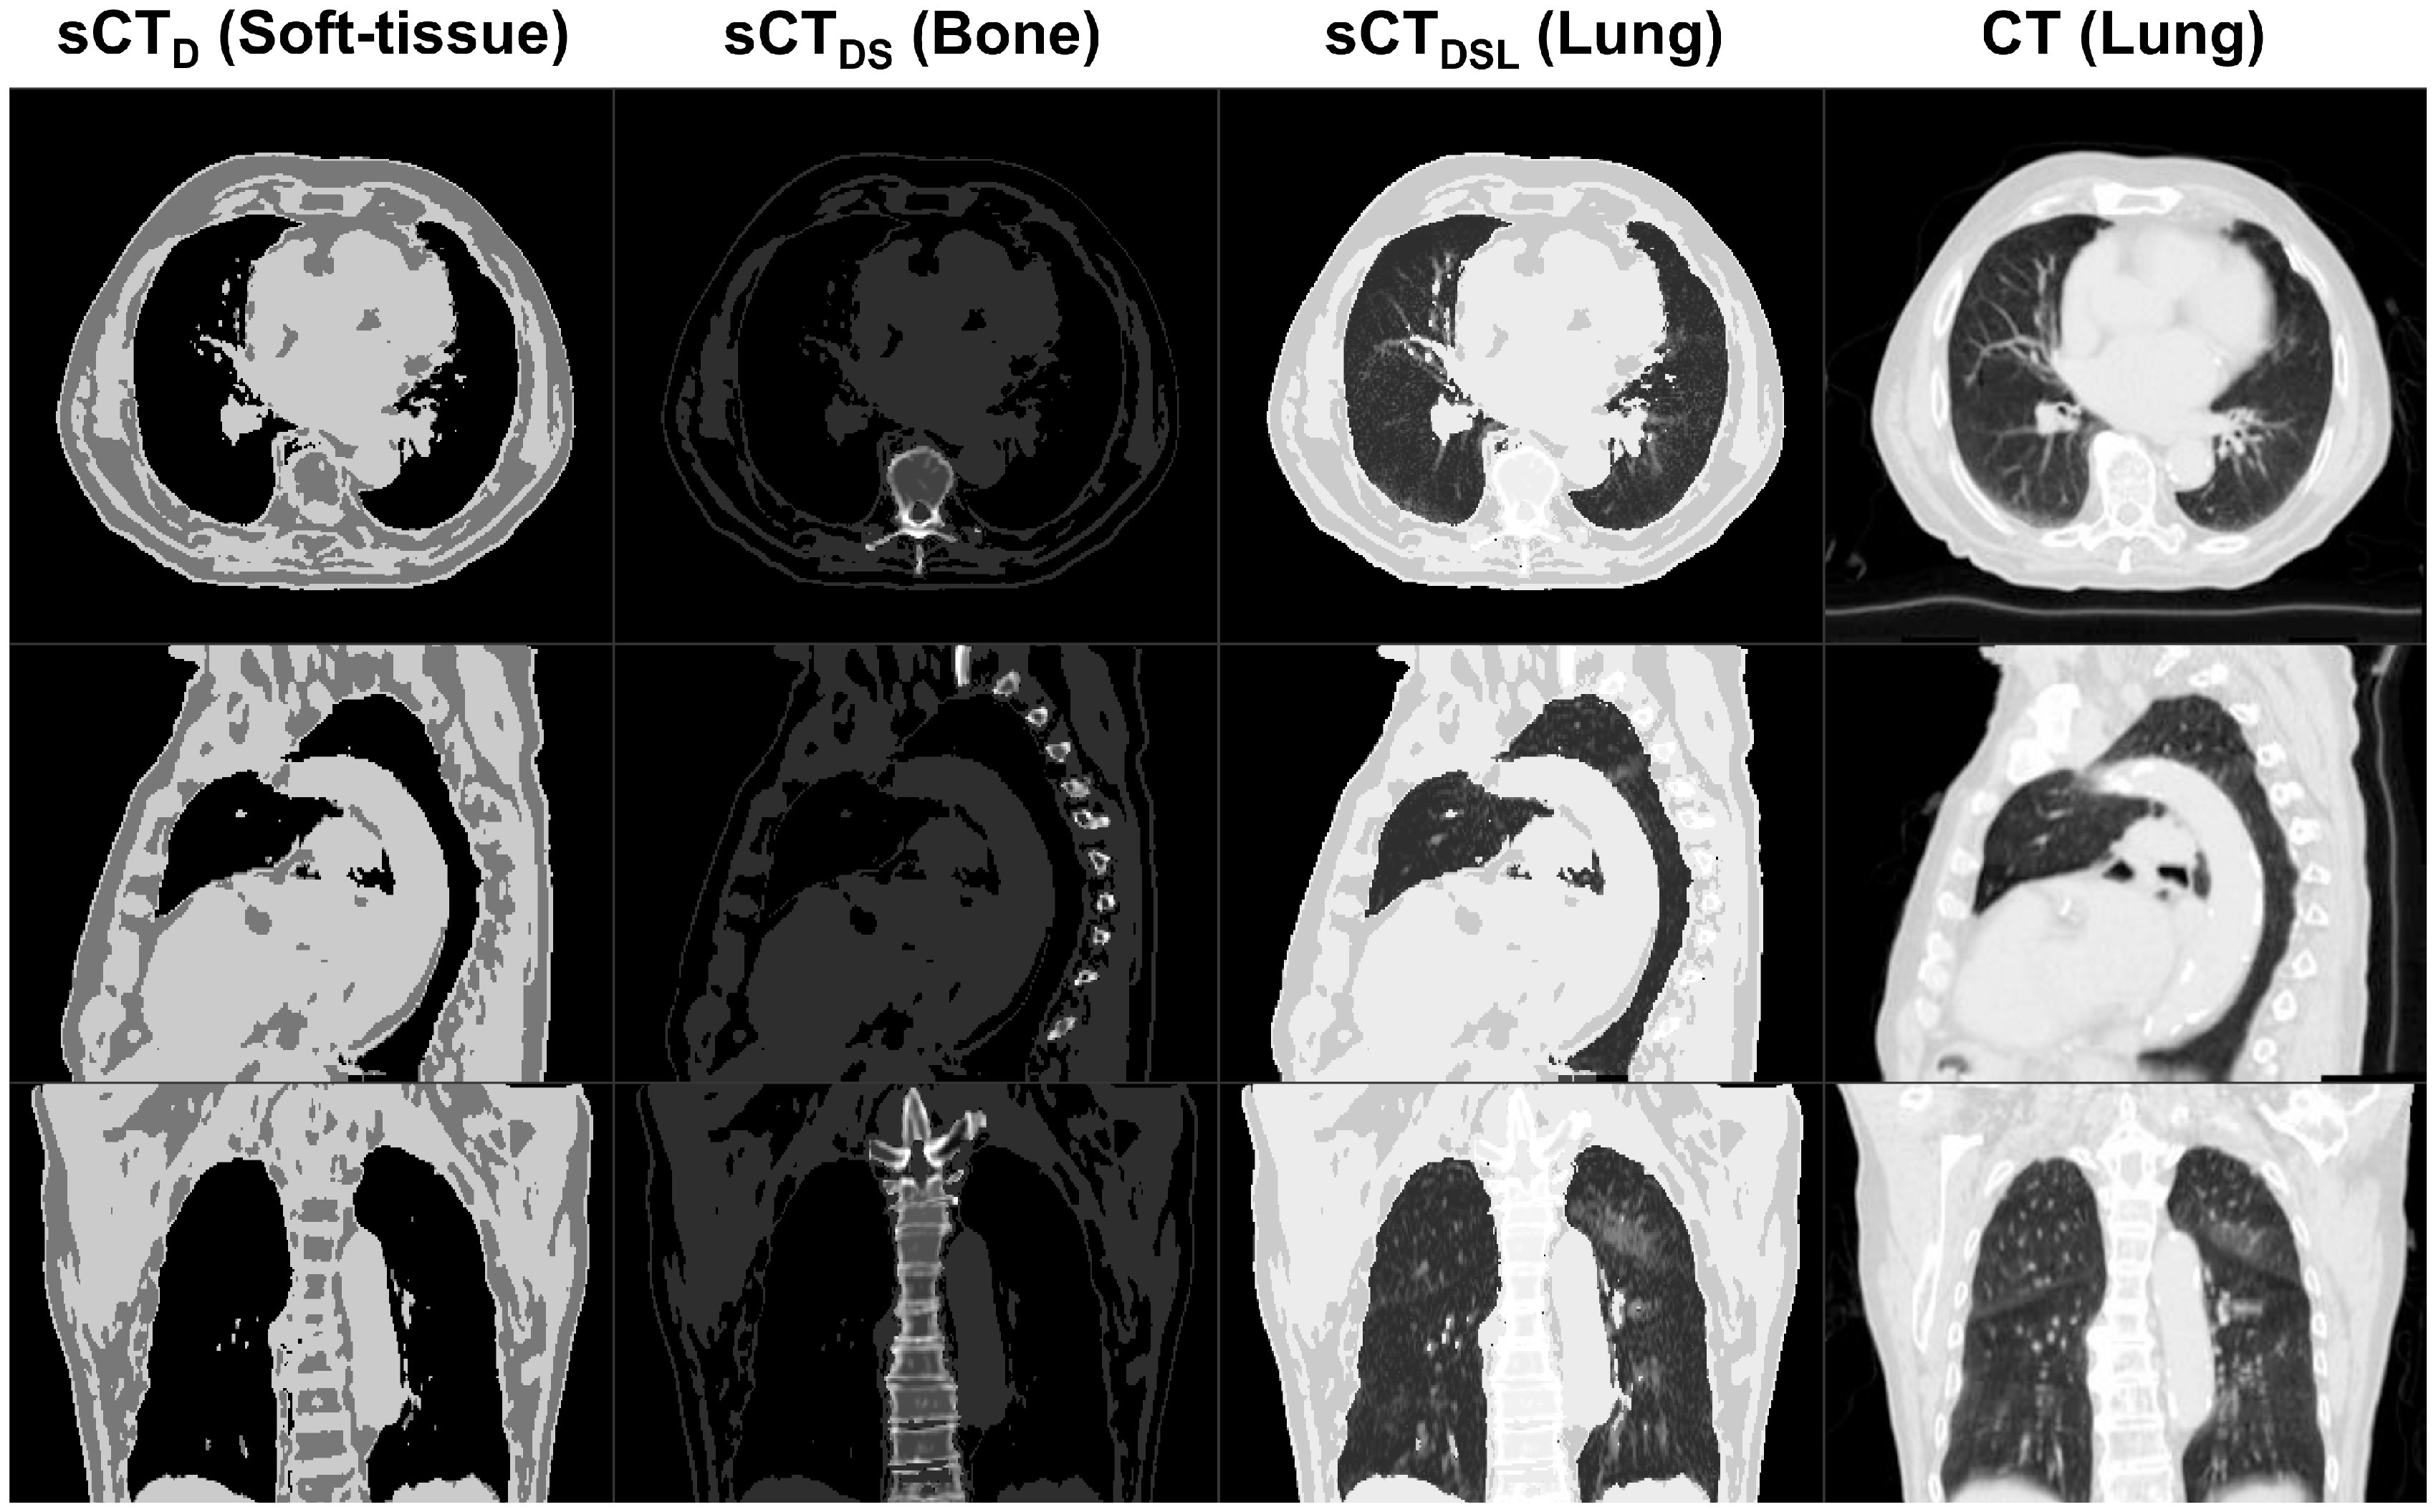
A comparison of Dixon (sCT_D_), Dixon-spine (sCT_DS_) and Dixon-spine-lung (sCT_DSL_) synthetic CT to midposition CT for patient 4. sCT is windowed (soft-tissue/bone/lung) to optimally display the new information that was introduced in each consecutive method.
